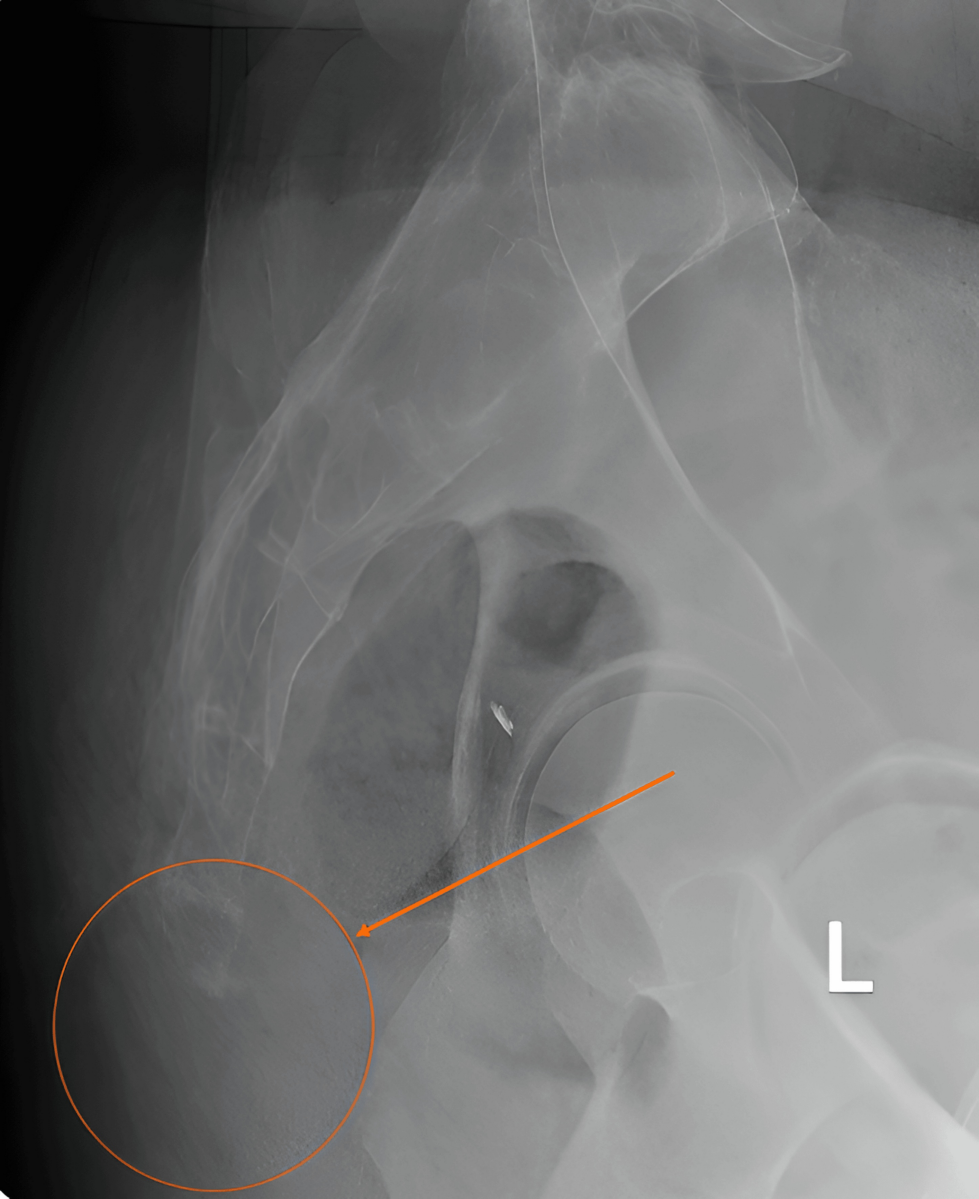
Postoperative lateral radiograph of the sacral-coccygeal region showing the five sacral bones with resected coccygeal segment (orange arrow)
